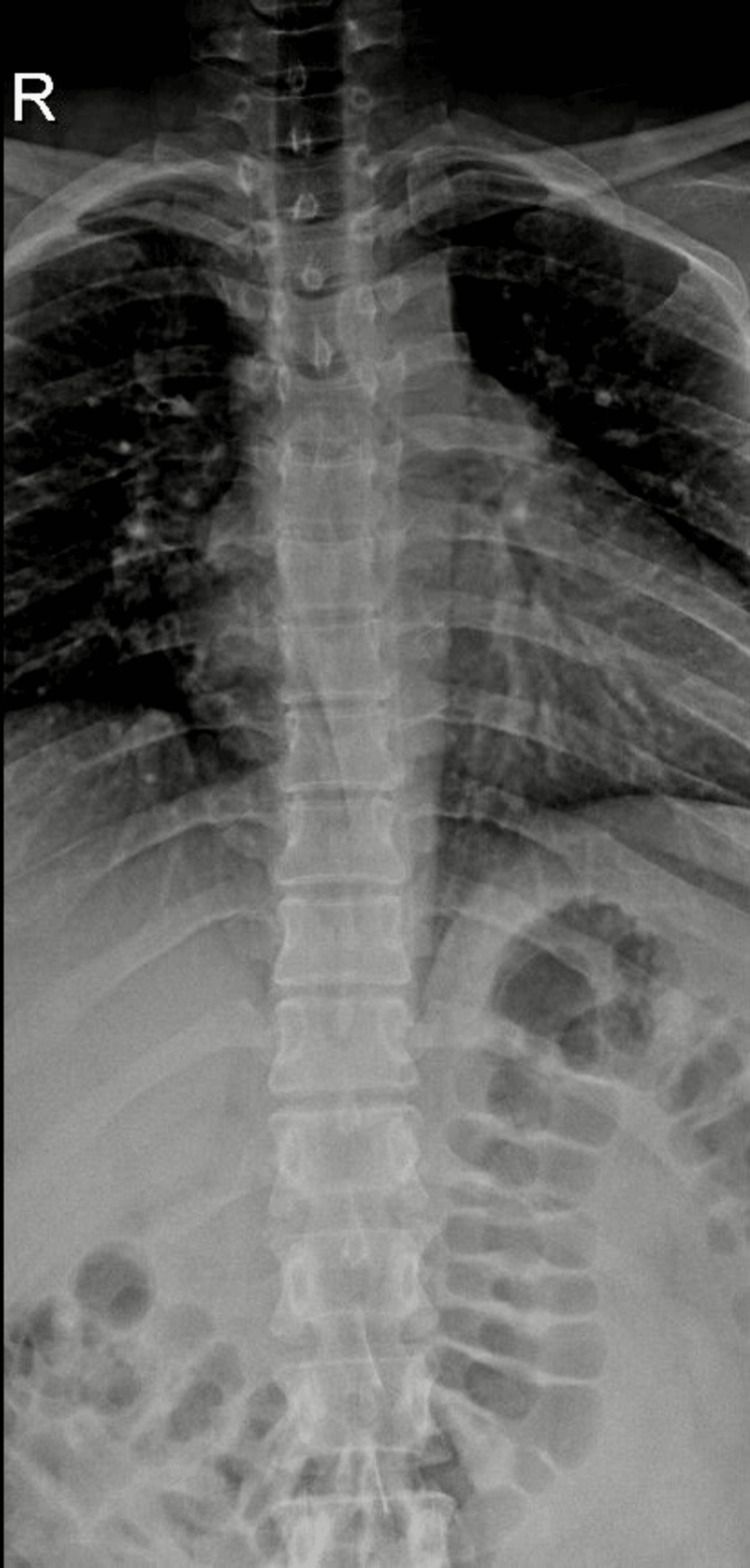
X-ray of the chest and abdomen
